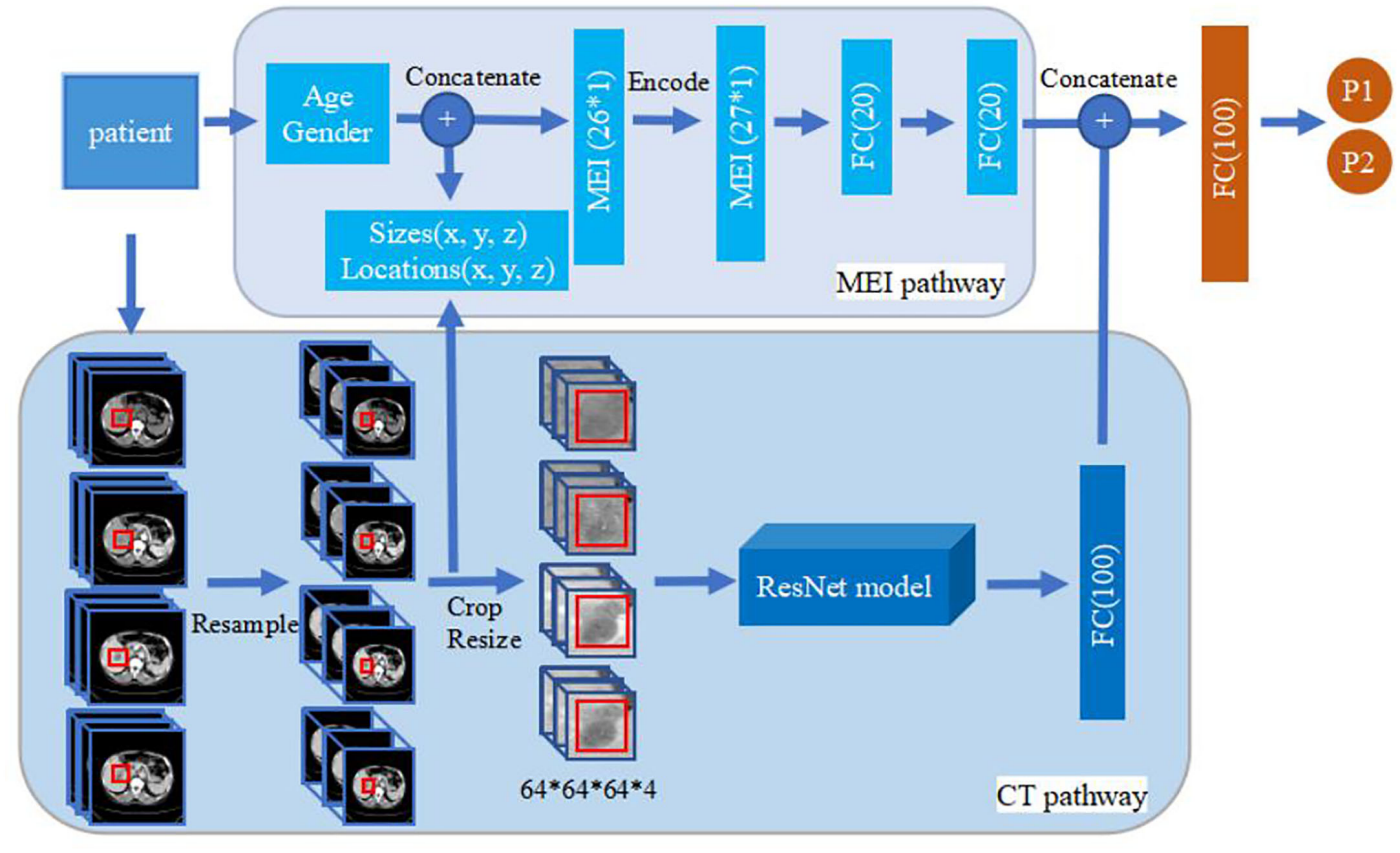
Overview of the proposed method. The upper part is MEI pathway and the lower part is the CT pathway. The 3D ResNet in CT pathway contains 14 layers (13 convolution layers, and 1 global average pooling layer). Filter size of the first convolution layer is 5×5×5, and the following filter sizes are 3×3×3. Filter size of the global average pooling layer is 2×2×2. The basic model and enhanced model only have the CT pathway. The size of image input in basic model is 64×64×64×1 while the others are 64×64×64×4. MEI, Minimum Extra Information.
